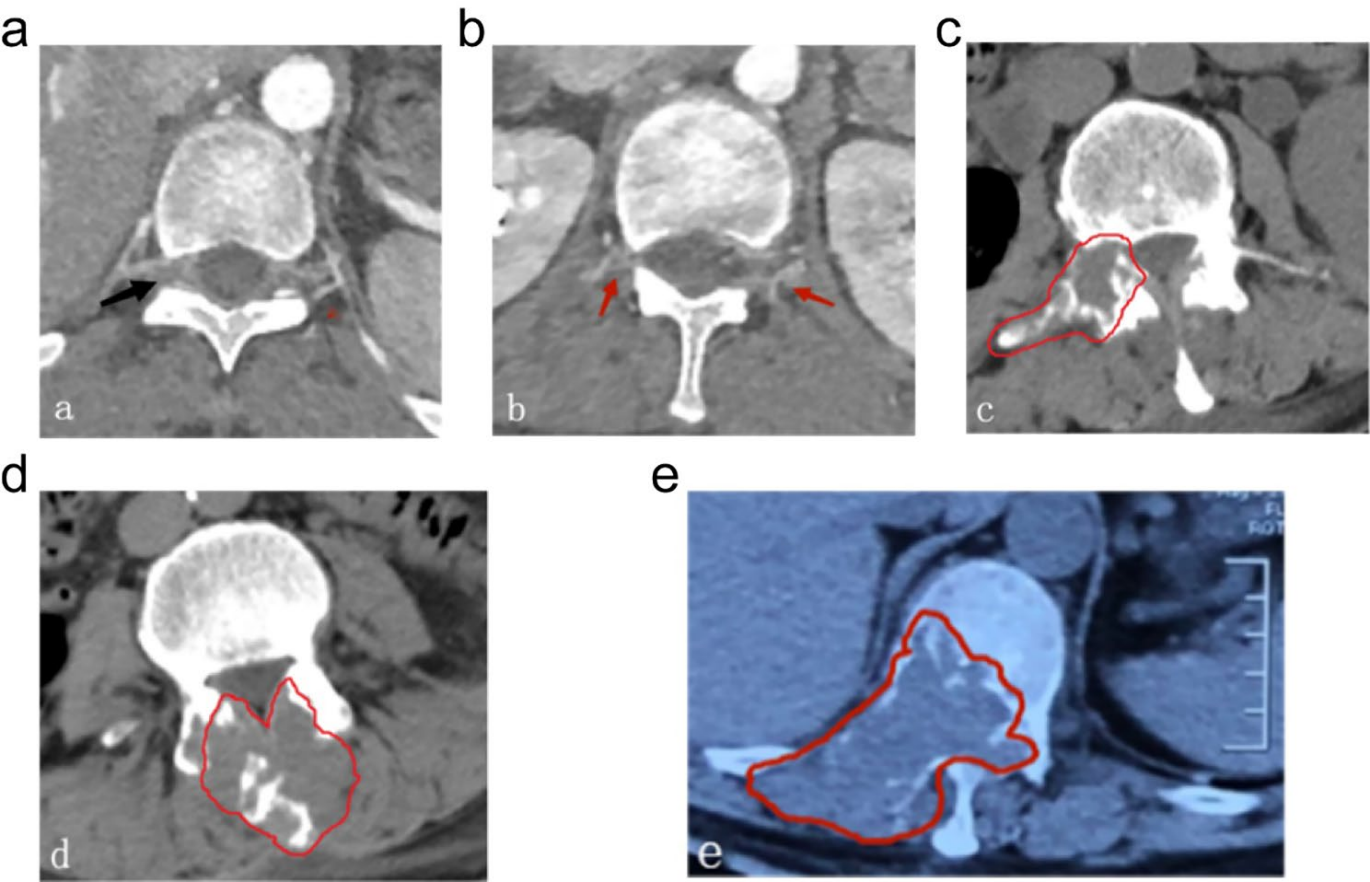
The vascular territory of the vertebral arch. (a) Enhanced CT showed that the right segmental artery-posterior branch-posterior branch of vertebral canal supplied blood posteriorly to the vertebral plate, spinal process, and ligamentum flavum (black arrow). (b) Enhanced CT showed that the bilateral segmental artery-dorsal branch-superior articular branch, inferior articular branch, and interarticular branch supplied blood to the superior and inferior articular processes, isthmus of pedicle, and pedicle. Bilateral segmental artery-transverse branch-transverse process anterior artery supplied blood to transverse process (red arrow). (c) CT displayed the areas of tumors in the right pedicles, transverse processes, and articular processes (red outline). (d) CT displayed the areas of tumors in the bilateral vertebral plates and spinal processes (red outline). (e) CT displayed the areas of tumors in the right vertebral body, pedicle, transverse process, joint process, and vertebral plate (red outline)
